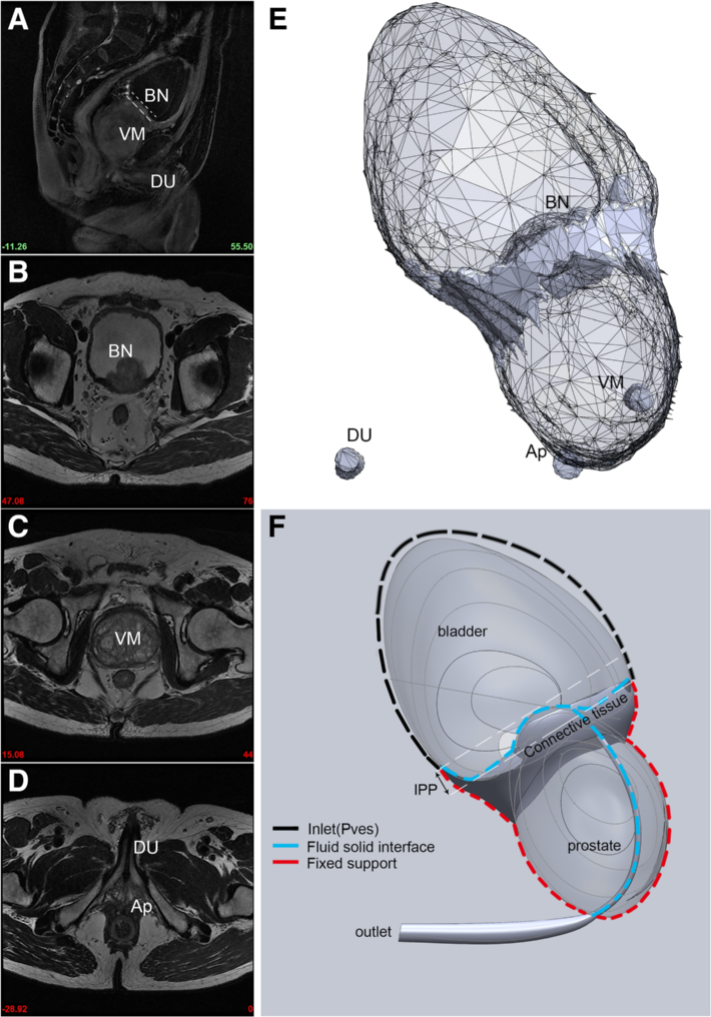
Prostate and bladder model construction MR images of lower urinary tract were collected (a sagittal plane, b-d axial plane, b bladder neck, c: veru montanum, d prostatic apex), and 3D model were reconstructed from organ contouring (e), and optimized (f). Measurement for IPP was shown in dash line in A and F. VM: veru montanum, BN: bladder neck, Ap: prostate apex, U: anterior urethra
